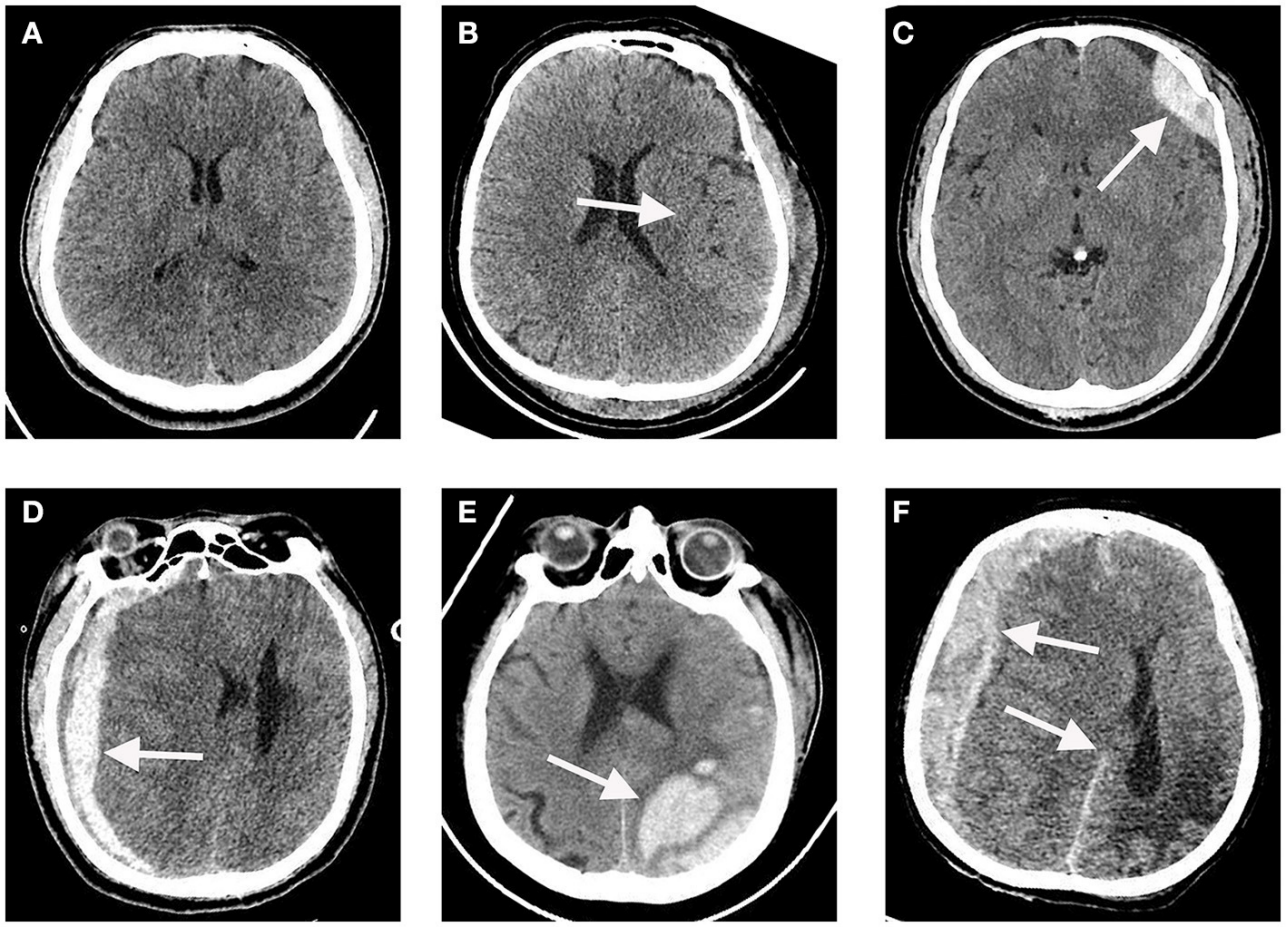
Representative CT images of a traumatic brain injury (TBI) and a normal CT scan. (A) Normal CT scan. (B) Cerebral contusion. (C) Extradural hematoma. (D) Subdural hematoma. (E) Subarachnoid hemorrhage. (F) Hemorrhage with midline shift. The white arrows indicate lesion regions.
